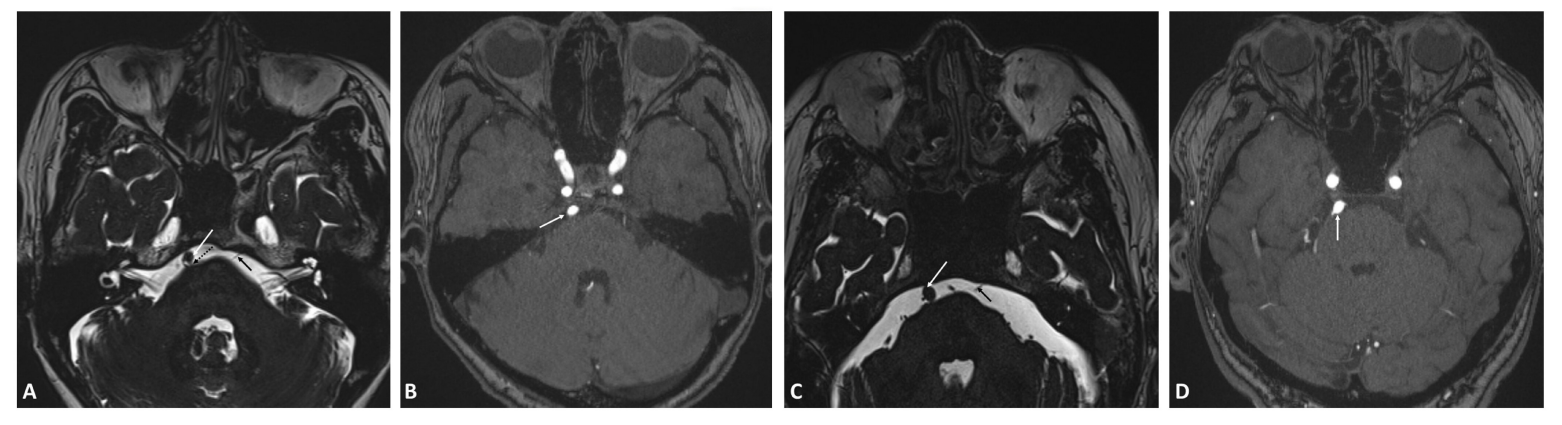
MRI images of case 1 (A,B) and case 2 (C,D). (A) and (C) are
